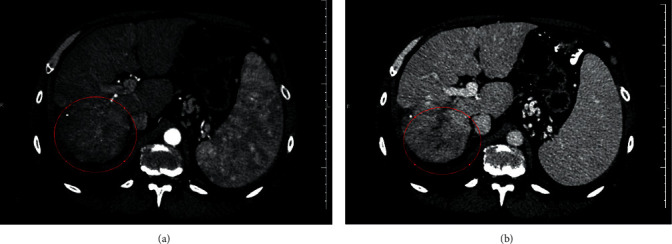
Computed tomography performed on October 1, 2018, showing the increase of the tumor dimensions. At this time, the tumor had 5.3 cm and still had arterial phase enhancement. It had led to thrombosis of the right portal vein branch. The committed segment is underscored by the red circle. (a) Arterial phase. (b) Portal phase.
